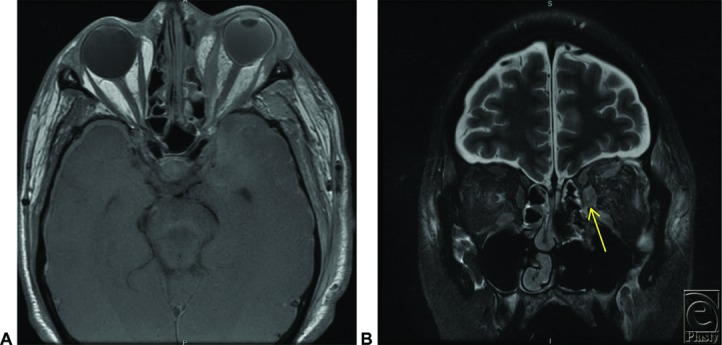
Orbital magnetic resonance image demonstrating left medial rectus involvement: (a) axial T1 SE; (b) coronal T2.
